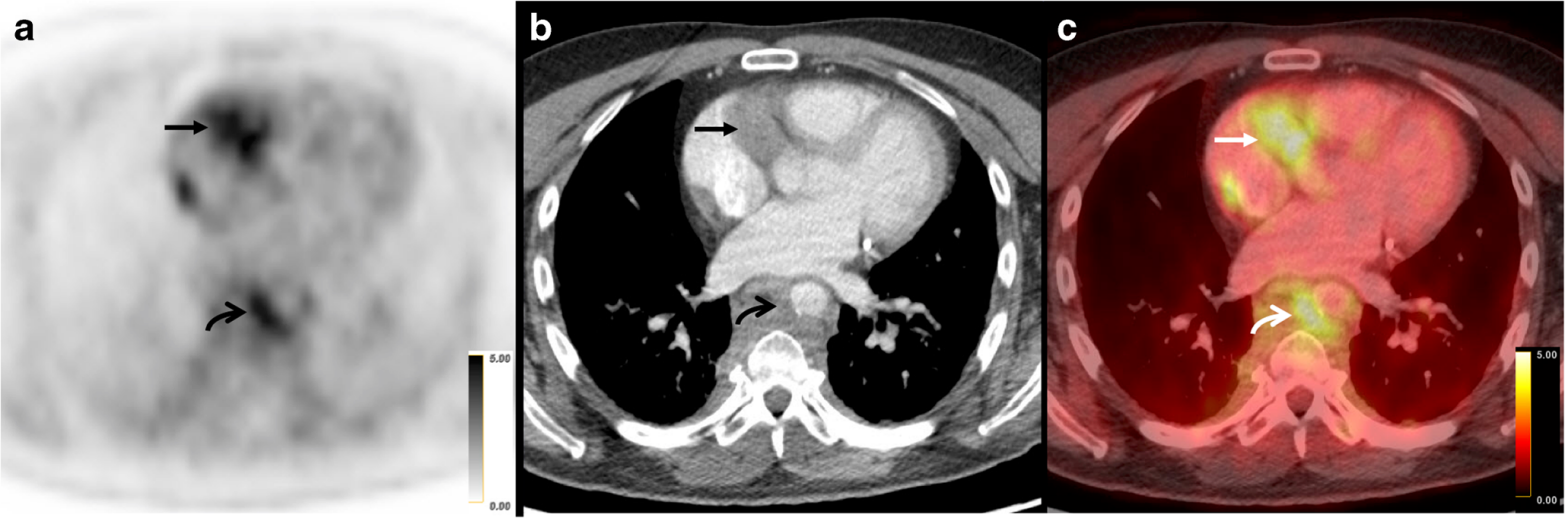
ECD detectable and measurable on both FDG PET and CT in a 53-year-old male. (a) Axial FDG PET, (b) axial CT, and (c) axial-fused FDG PET/CT through the level of the heart demonstrate FDG-avid cardiac soft tissue with a SUV_max_ of 4.2 (arrows), as well as FDG-avid peri-aortic soft tissue with a SUV_max_ of 4.0 (curved arrows)
